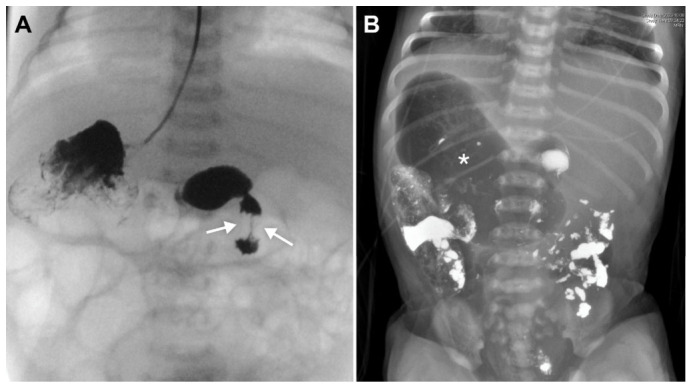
(A) Upper gastrointestinal series of a female neonate with situs anomaly demonstrating the site of duodenal obstruction, indicated by a white arrow, on the fourth day of life. (B) Post-upper gastrointestinal series abdominal radiograph of the same patient showing a mirror-image distended stomach, marked by an asterisk (*), and left-sided duodenum on the third day of life.
